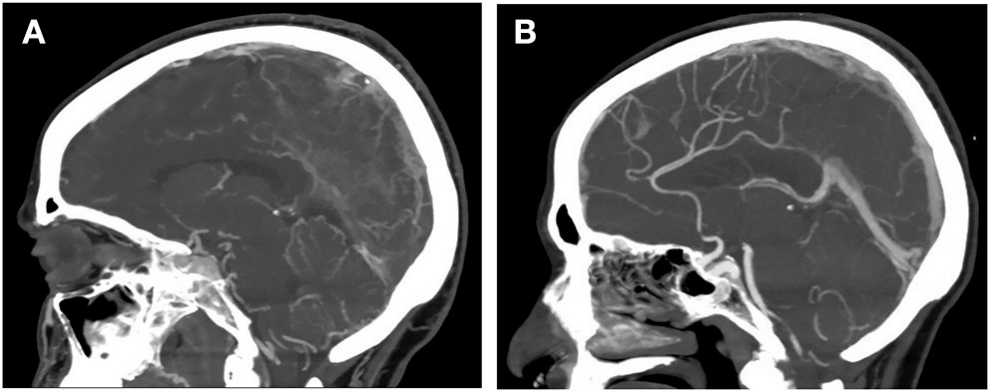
CT cerebral venography showing massive cerebral venous thrombosis associated with COVID-19 infection. (A) At admission. (B) After 3 weeks of anticoagulation therapy.
